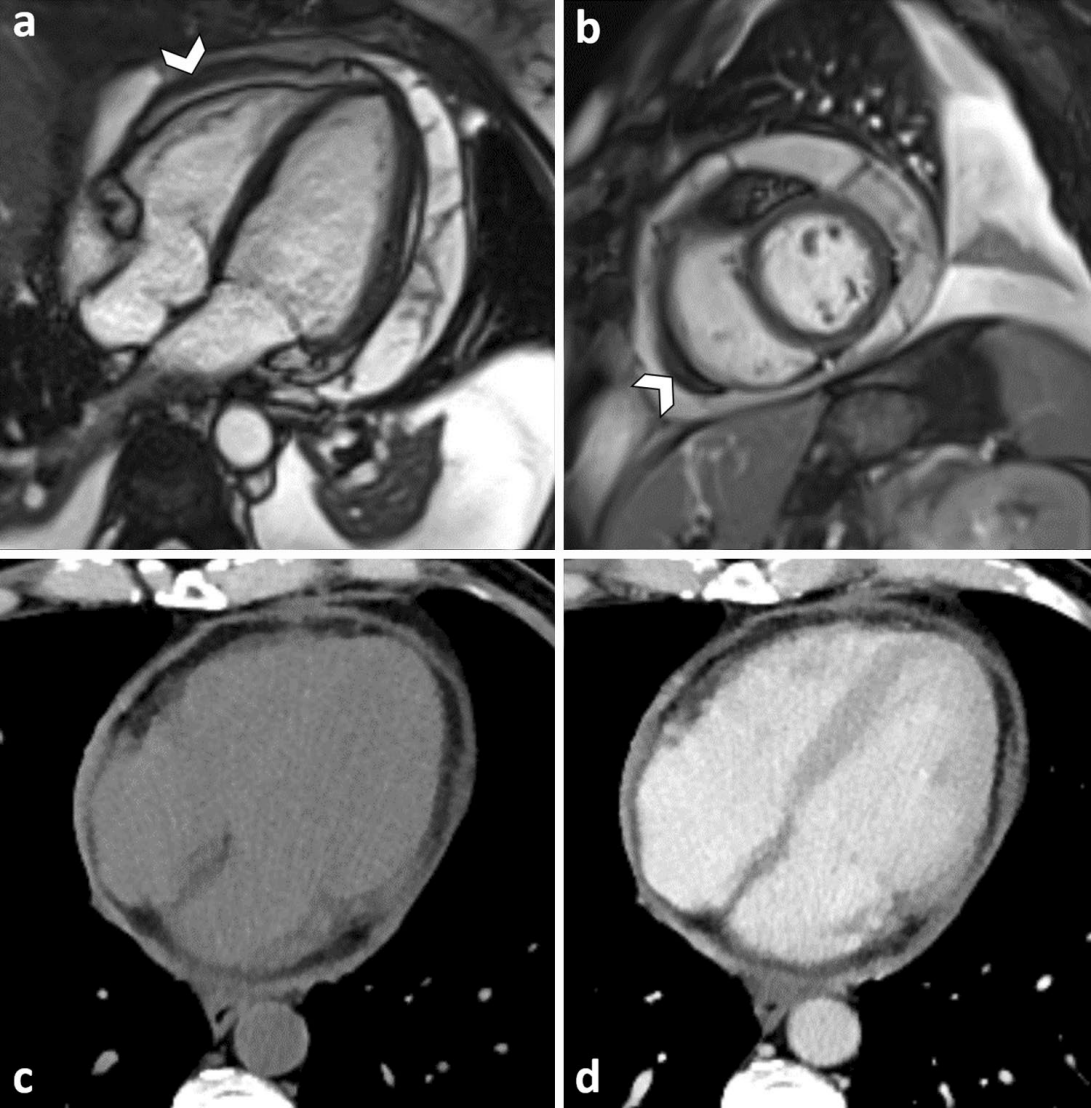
Cine MRI scan on 4-chamber (a) and short-axis (b) view, shows circumferential pericardial effusion with multiple linear septa within the pericardial sac, indicating fibrin deposition. Note thickening of the visceral pericardium (arrowheads). CT before (c) and after contrast (d) shows diffuse thickening and enhancement of the pericardium in a patient affected by lung carcinoma
